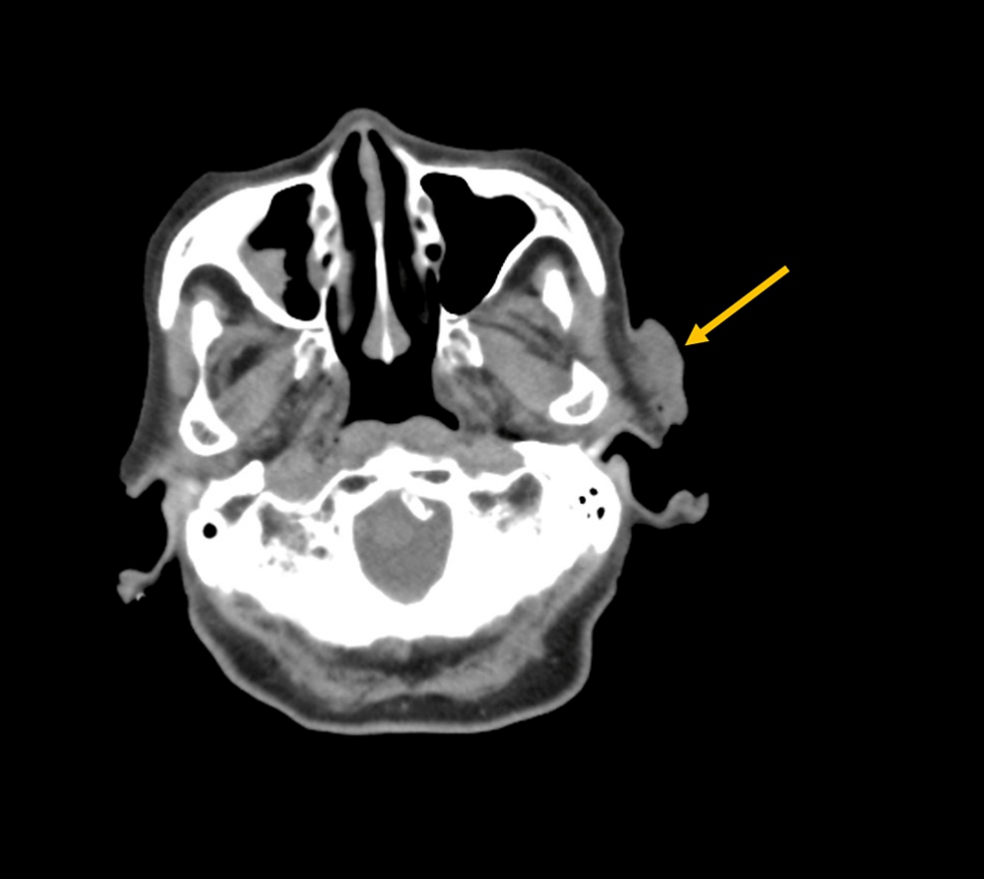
Computed tomography image obtained before radiotherapy. The arrow shows a tumor.
